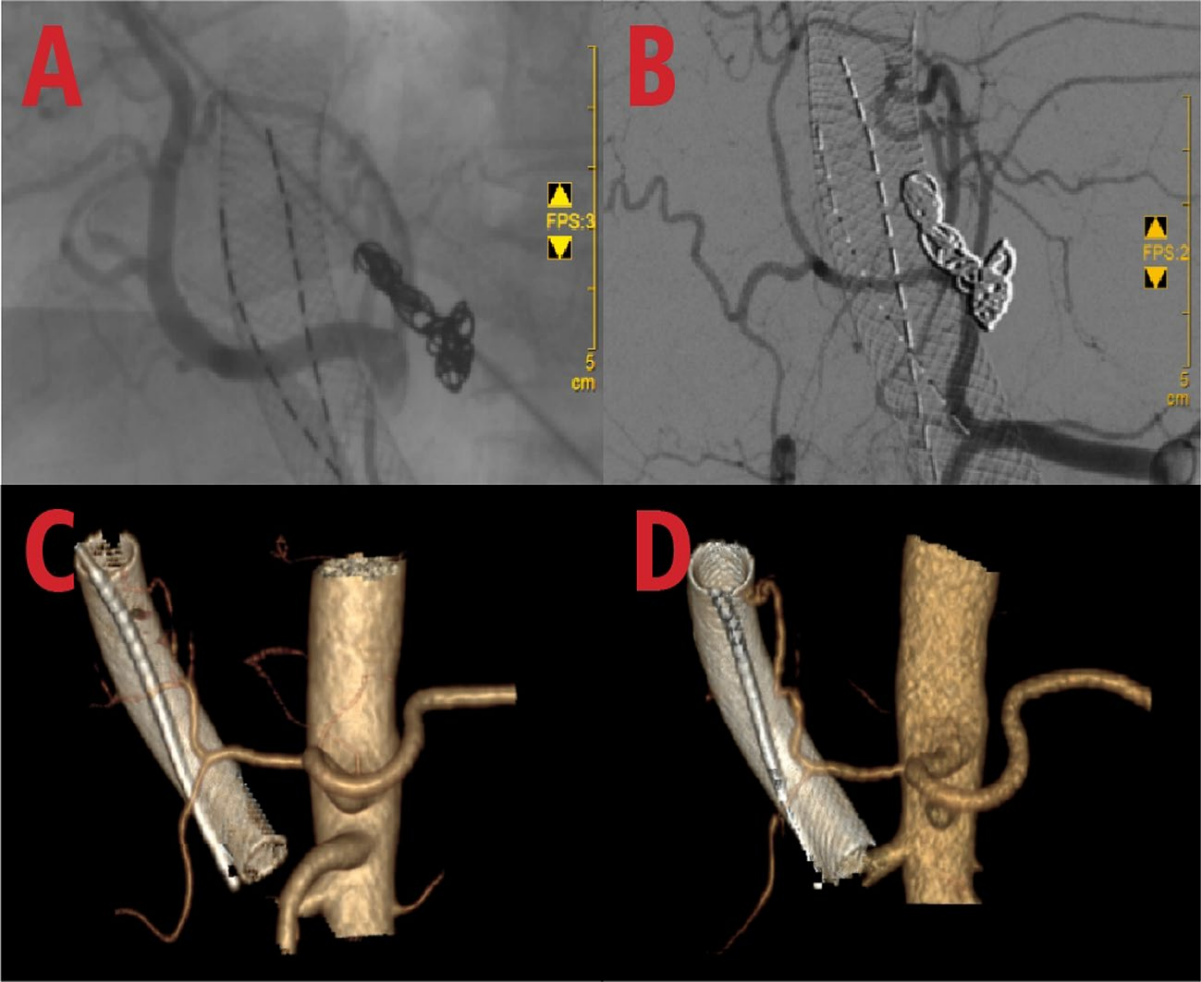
Hepatic artery angiography at 1 months (A), 3 months (B) and CT scan at 5 months (C), 7 months (D) after stent implantation showing a gradual decrease in the diameter of the hepatic artery. After stent implantation showing a gradual decrease in the diameter of the hepatic artery
